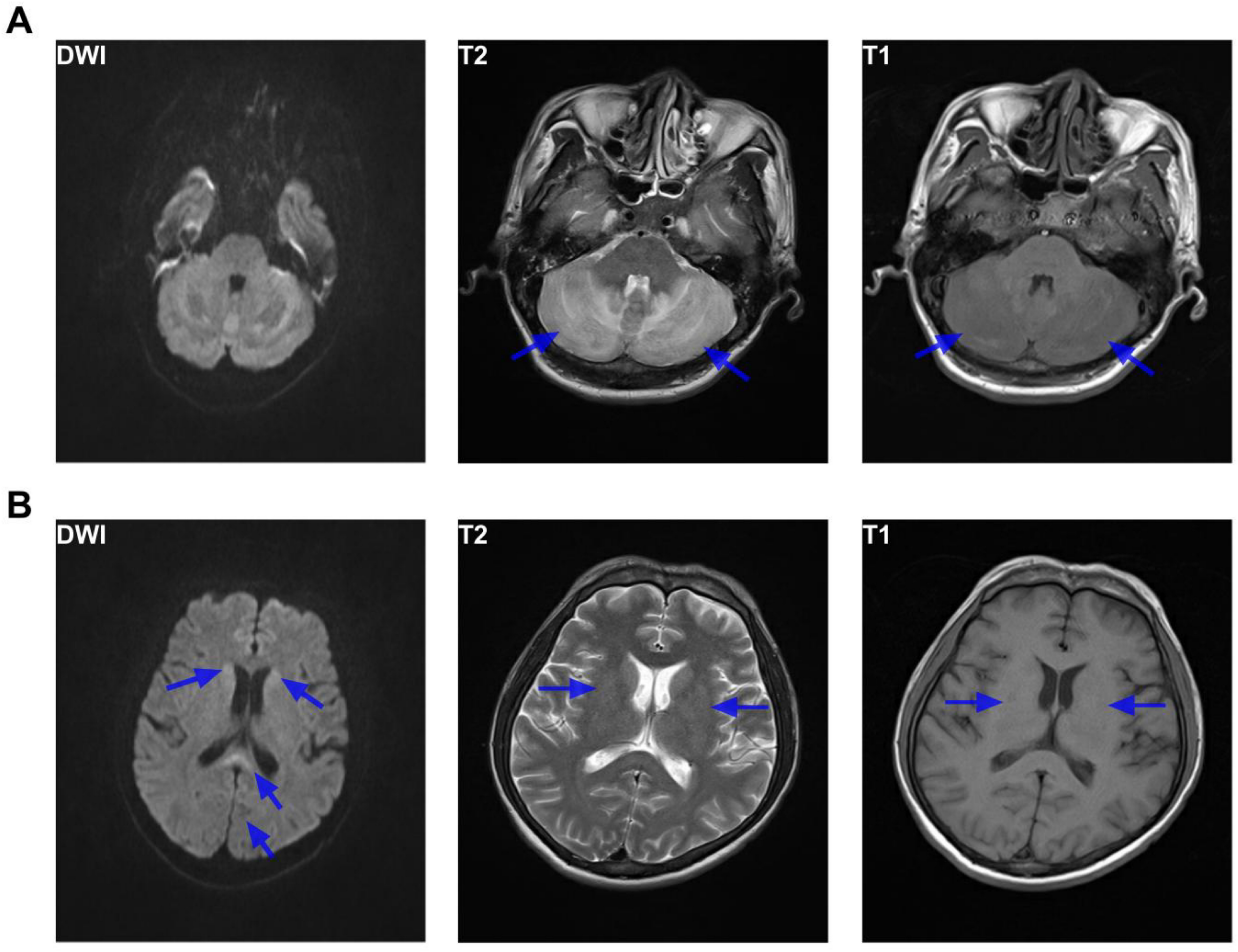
Brain MRI findings (day 12). Brain MRI showing (A) patchy mixed T1- and T2-weighted signal abnormalities in the bilateral cerebellar hemispheres, and (B) patchy, slightly hyperintense T2-weighted signals in the bilateral basal ganglia. DWI demonstrates small foci of restricted diffusion in the splenium of the corpus callosum, bilateral caudate heads, and left temporo-occipital watershed region, as indicated by the blue arrows.
